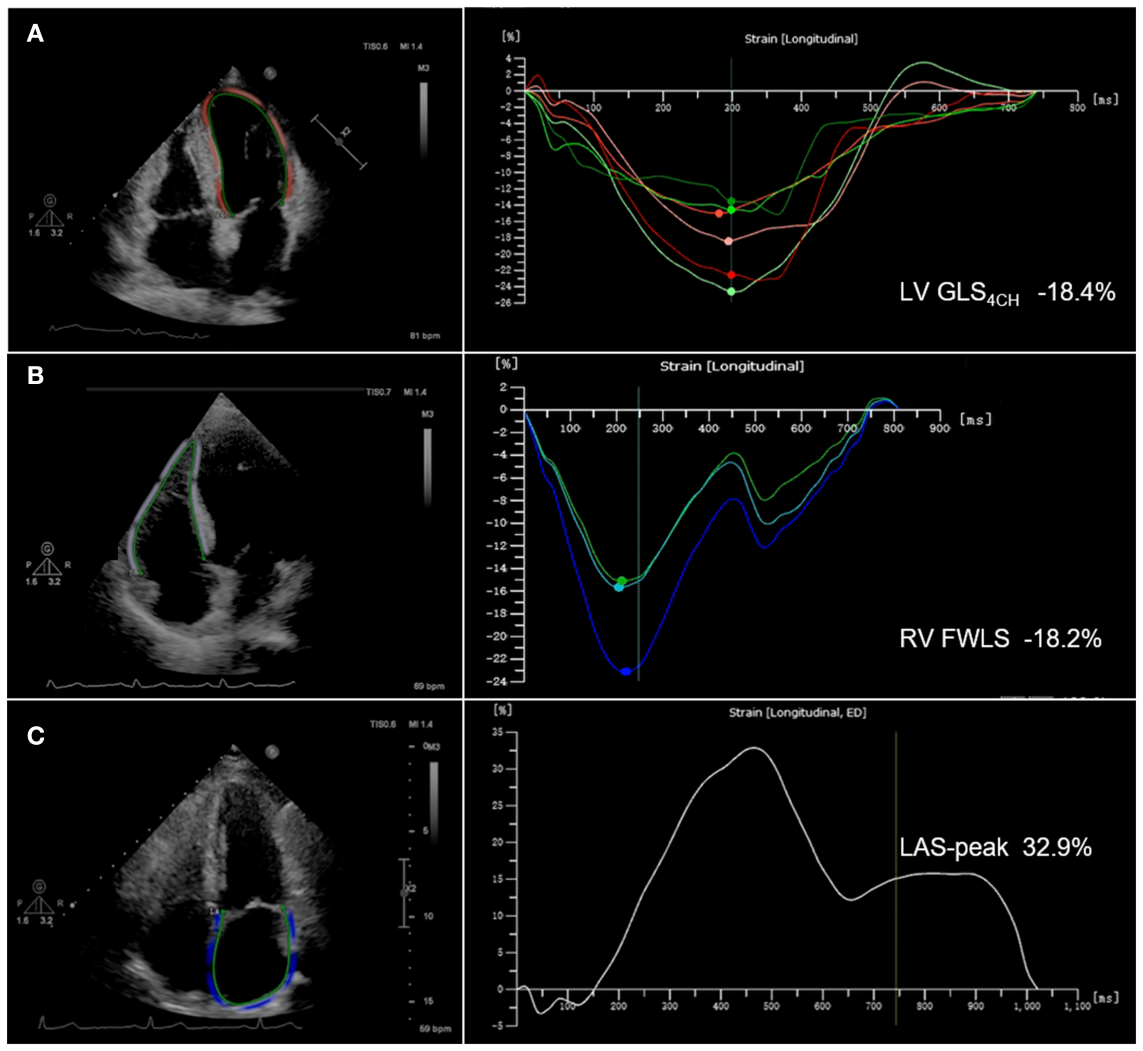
Biventricular and left atrial longitudinal strain obtained from two-dimensional speckle-tracking echocardiography in COVID-19 patients. (A) Representative image with left ventricular global longitudinal strain from the apical 4-chamber view (LV GLS_4CH_); (B) Representative image with right ventricular free wall longitudinal strain (RV FWLS); (C) Representative image with peak left atrial strain (LAS-peak).
